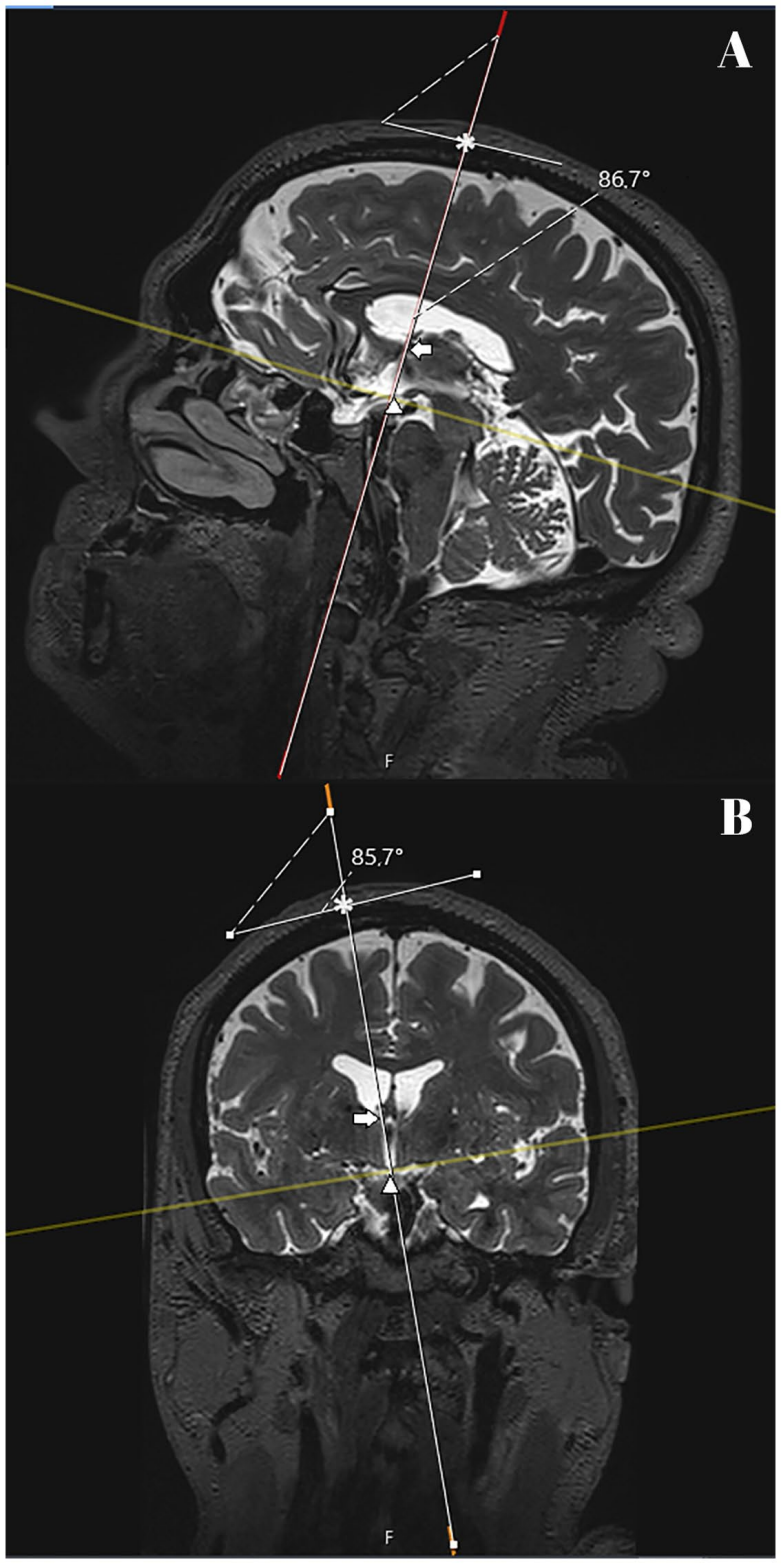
T2 SPACE MRI imaging showing sagittal (A) and coronal (B) views of the brain hemispheres of a non-hydrocephalic patient with Parkinson’s disease. The full white line represents the delineated pathway of the neuroendoscope for each of the patients. A tangential plane to the entry point was drawn and intersected with the pathway line, marking the upper limit of the pathway (*). The arrow marks the point of intersection of the pathway with the interventricular foramen (of Monro). The tip of the arrowhead marks the intersection between the pathway line and its lower limit, the tuber cinereum. The angulation between the plane tangential to the entry point and the pathway was measured to verify the proximity of the angle to 90° in each patient. The dashed line between the intersecting full lines indicates the side at which the angle was measured. In this patient, the anterior angulation was 86.7° in the sagittal plane (A) and the lateral was 85.7° in the coronal plane.
